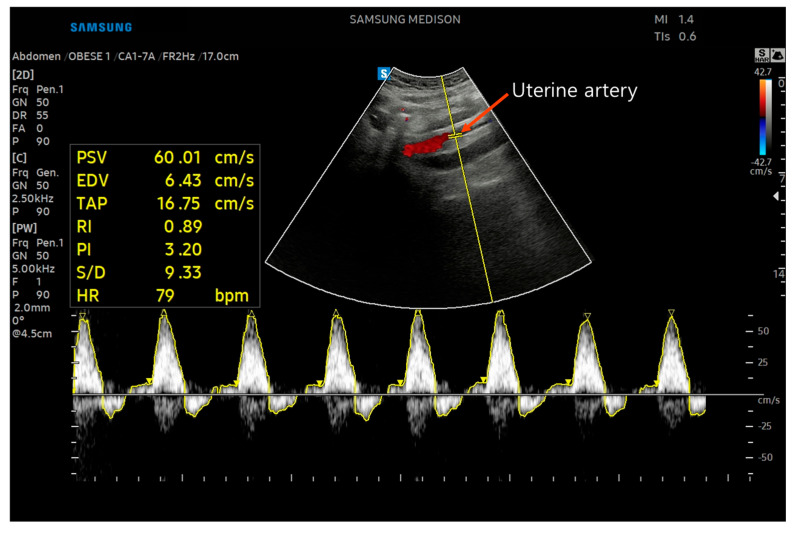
Doppler ultrasound measurement of uterine artery pulsatility index, resistive index.
